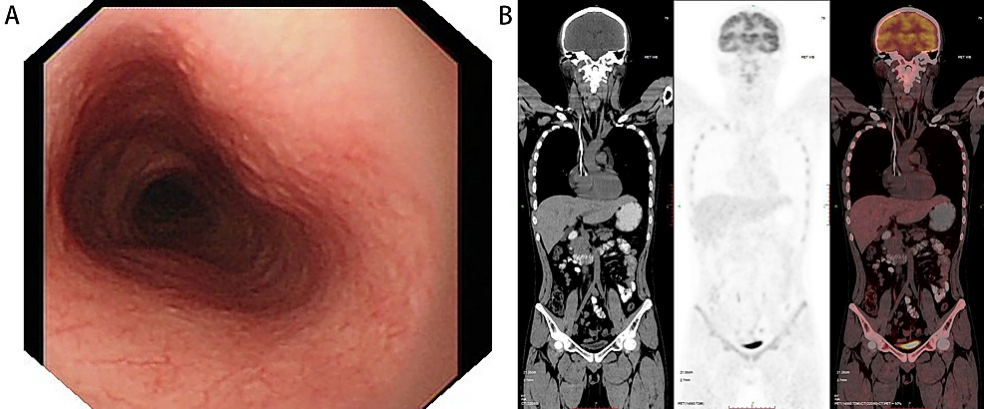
Follow-up of primary esophageal lymphoma after eight courses of CHOP regimen chemotherapy (A) Gastroscopy showed that the original esophageal tumor disappeared completely, the mucous membrane of each segment of the esophagus was smooth, there was no ulcer and esophageal stricture. (B) PET-CT showed that there was no obvious abnormality in glucose metabolism in all segments of esophagus, no metastatic hypermetabolic foci were found in paraesophageal lymph nodes, mediastinal lymph nodes, and other parts of the body, and no tumor recurrence and metastasis were found.
